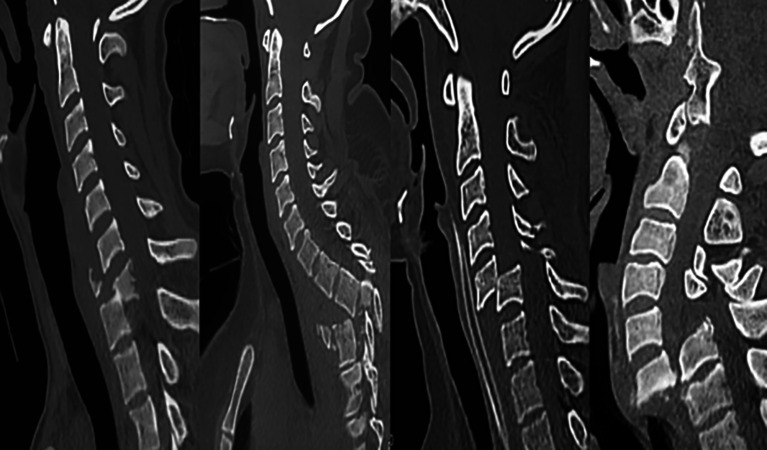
CT images of spondyloptosis at various anatomical levels of spine.
